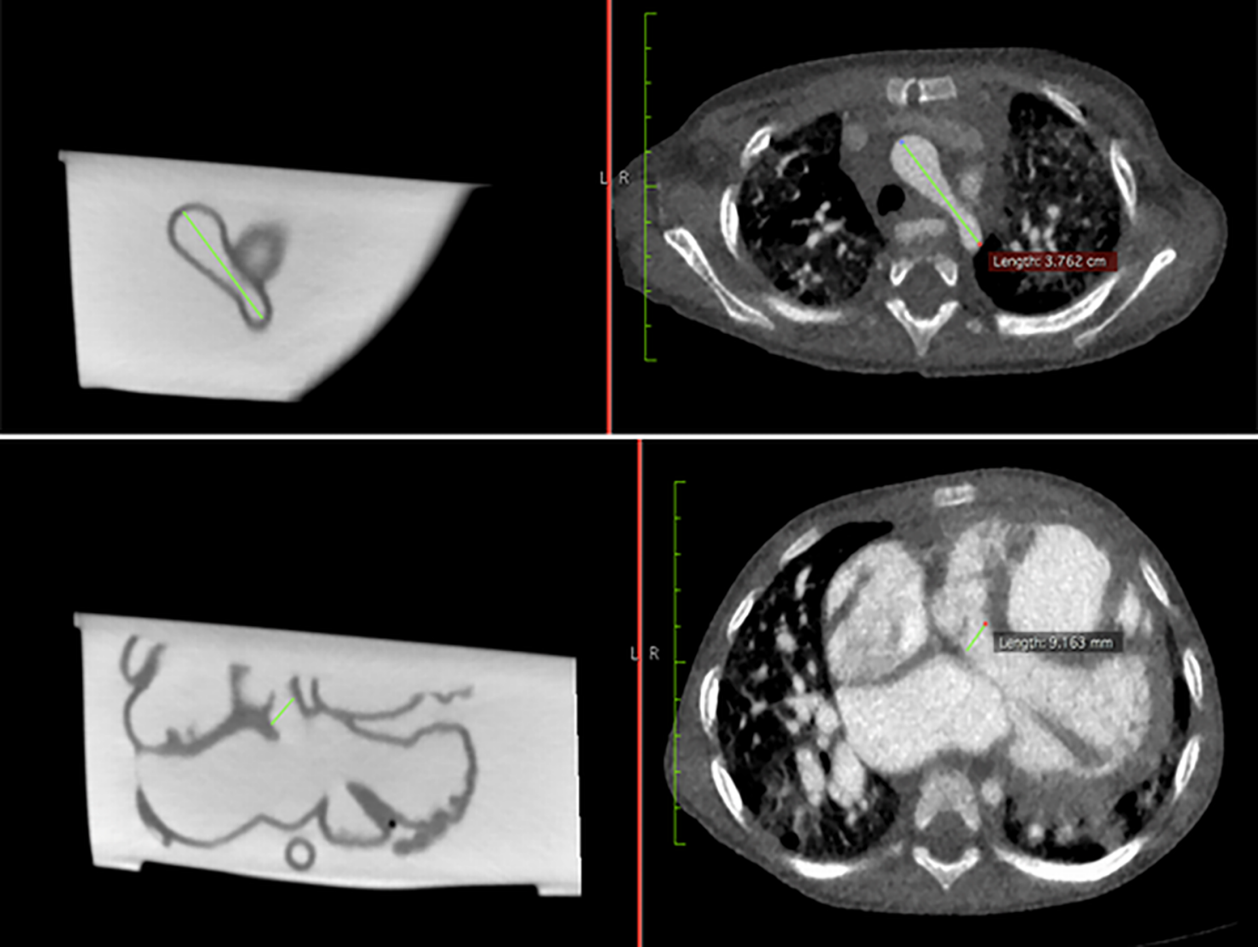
An example showing how measurements were taken at the aortic arch (top row) and ventricular septal defect (bottom row) using ruler function in *Horos*. The left column images refer to contrast-enhanced CT images of the 3D printed model, while the right column images indicate original CCTA images.
